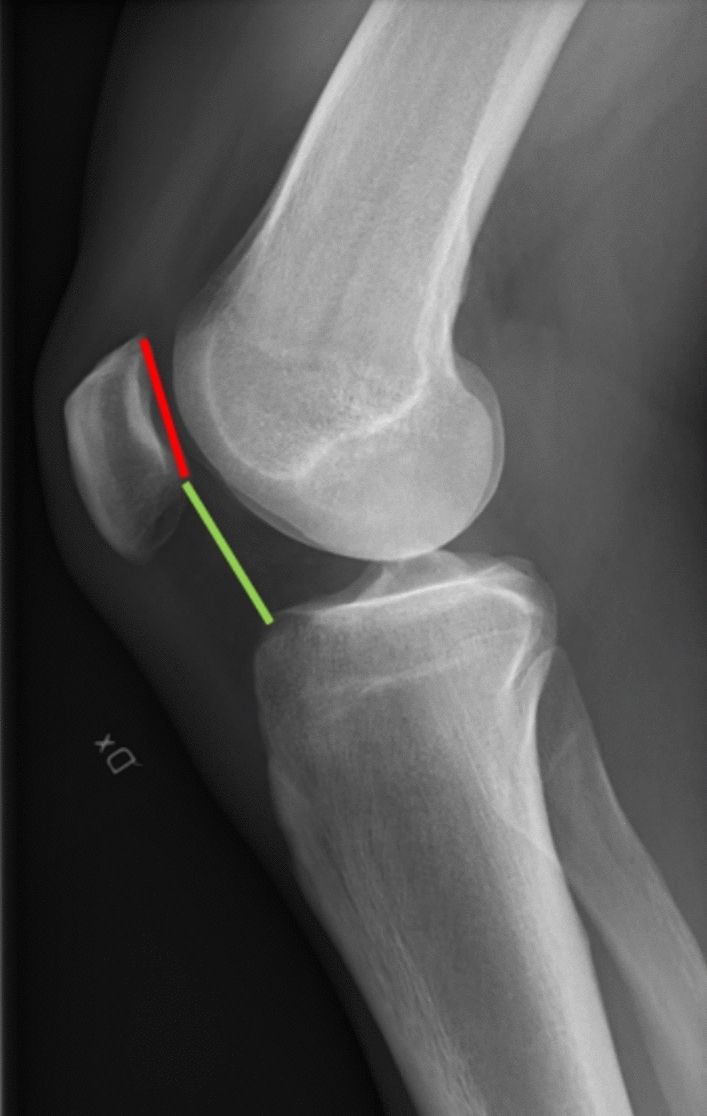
Caton-Deschamp index measured on a lateral X-ray. The distance between the anterior aspect of the tibial plateau and the most inferior aspect of the patellar articular surface (green line) is divide by the length of the cartilaginous articular surface of the patella (red line). Normal values are between 0.6 and 1.2
